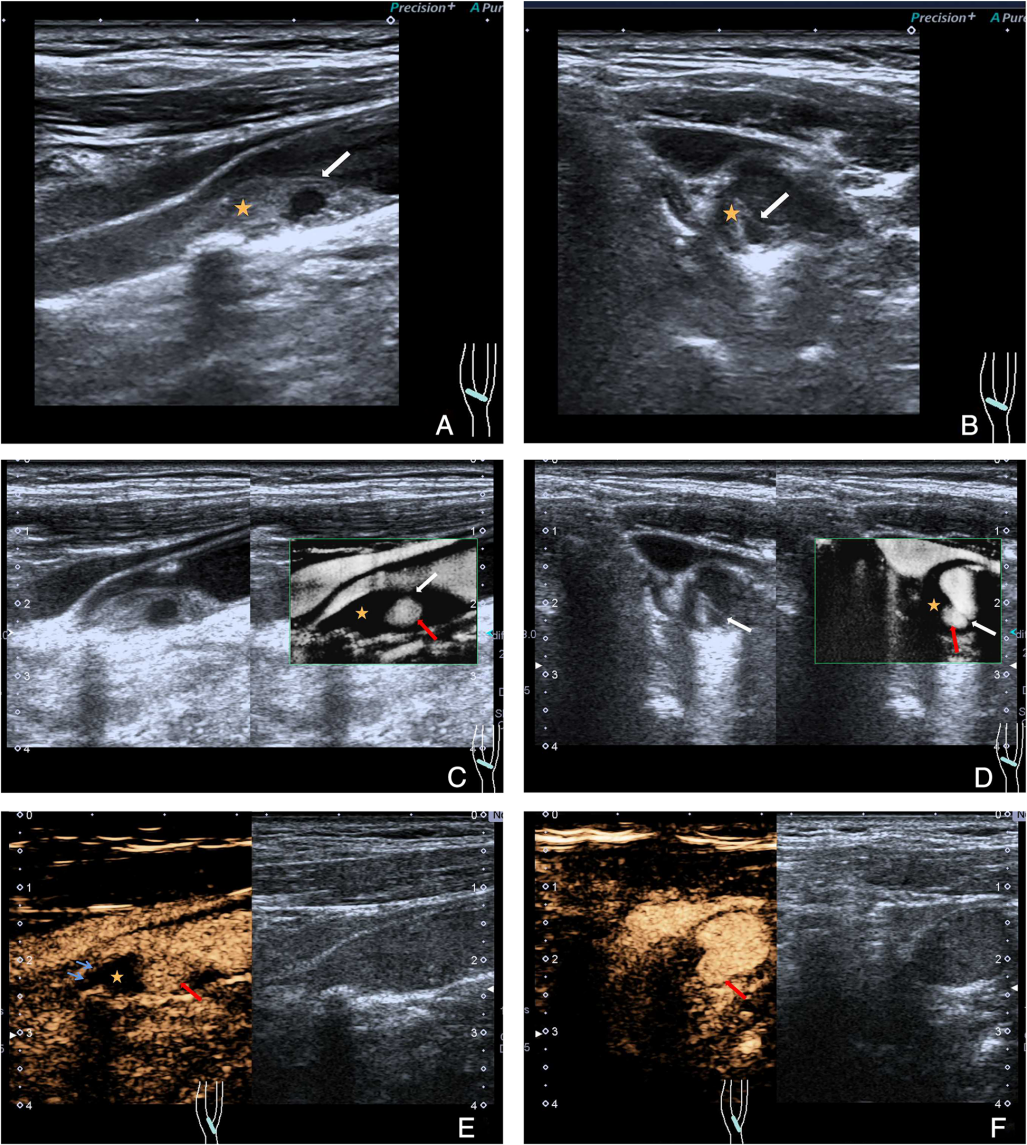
Multimodal ultrasound features of a carotid web (white arrow) grows along the long axis of the plaque (orange star) which locates on the posterior wall of the right carotid artery bifurcation. A, B B-mode ultrasound shows a mixed isoechoic plaque with a membrane-like structure and a cavity. C, D Superb microvascular imaging reveals cliff-like defect on the longitudinal plane and liner defect on the cross-section. E, F Contrast-enhanced ultrasound shows contrast agents entering the cavity (red arrow), with plaque enhancement (grade 1, blue arrows)
